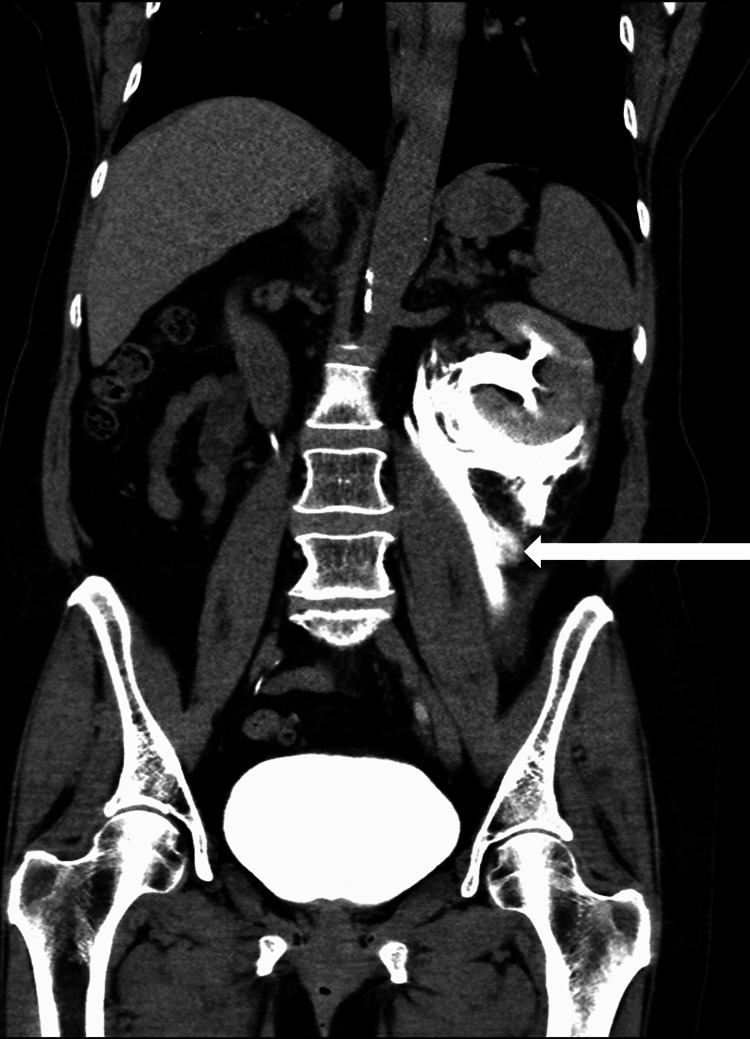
Delayed post-contrast CT scan revealing contrast extravasation from the left ureter (white arrow).
